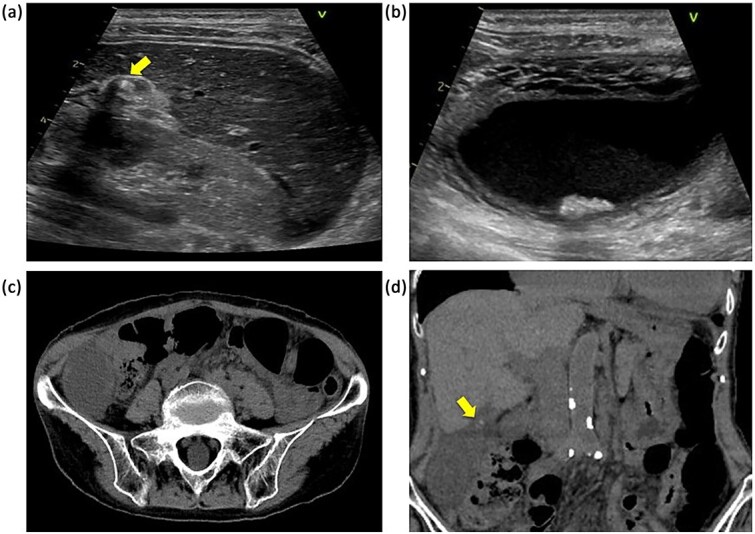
Abdominal ultrasonography and CT findings. (a) Impacted gallstone in the gallbladder neck (arrow). (b) Enlarged gallbladder and edematous thickening of the gallbladder wall. (c) Enlarged gallbladder in the right lower abdominal quadrant. (d) Enlarged gallbladder and gallstone in the neck of the gallbladder (arrow).
